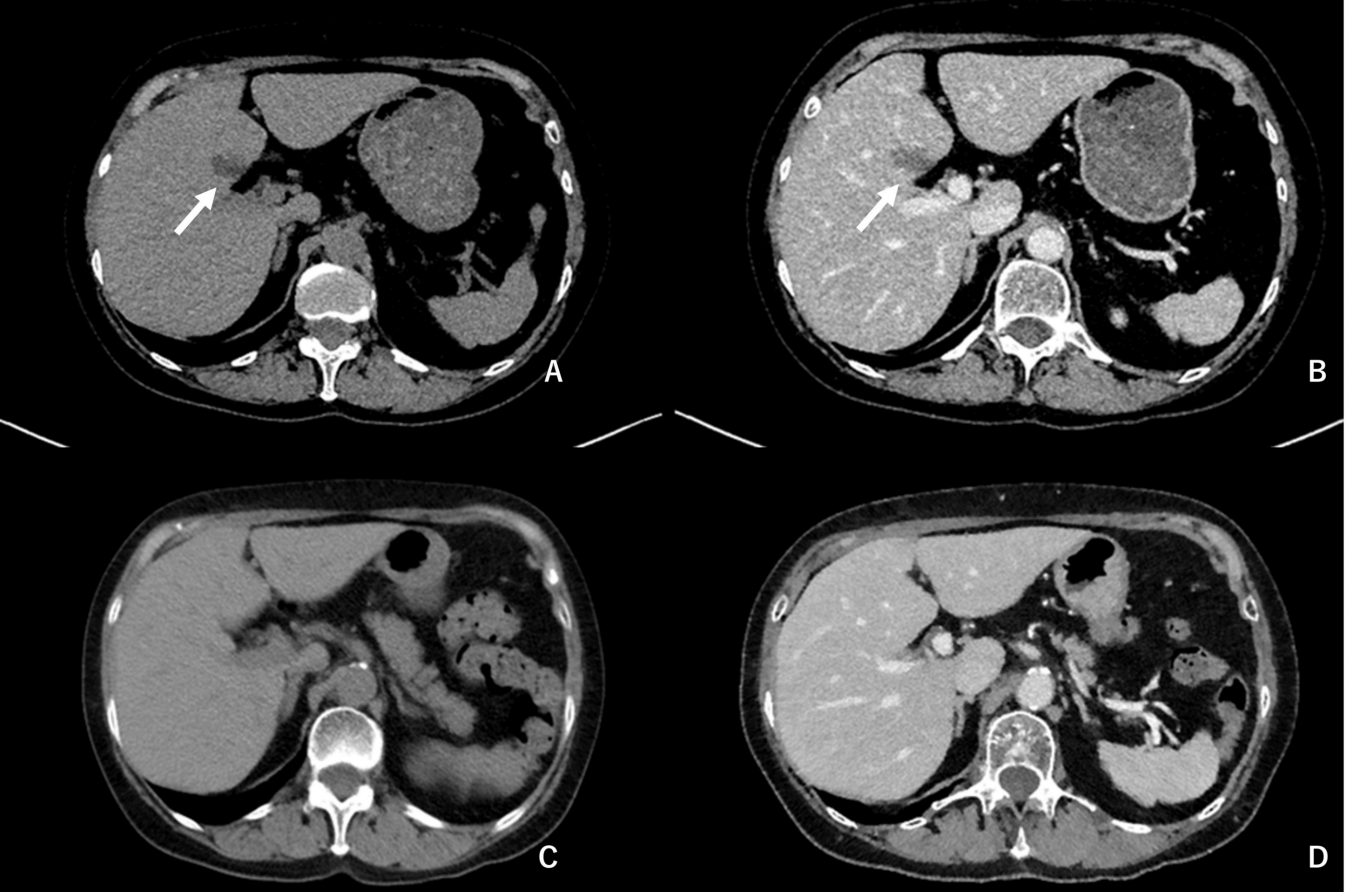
CT findings of the S4 steatotic pseudolesion A: non-contrast CT at presentation shows an oval hypoattenuating lesion (approximately 11 × 23 mm) in segment IV (S4) near the hepatic hilum (arrow); B: contrast-enhanced CT at presentation shows a corresponding hypoenhancing nodule (arrow); C: non-contrast CT three months after treatment initiation shows complete resolution of the lesion; D: contrast-enhanced CT at the same time point confirms disappearance of the lesion. CT: computed tomography; S4: segment IV
